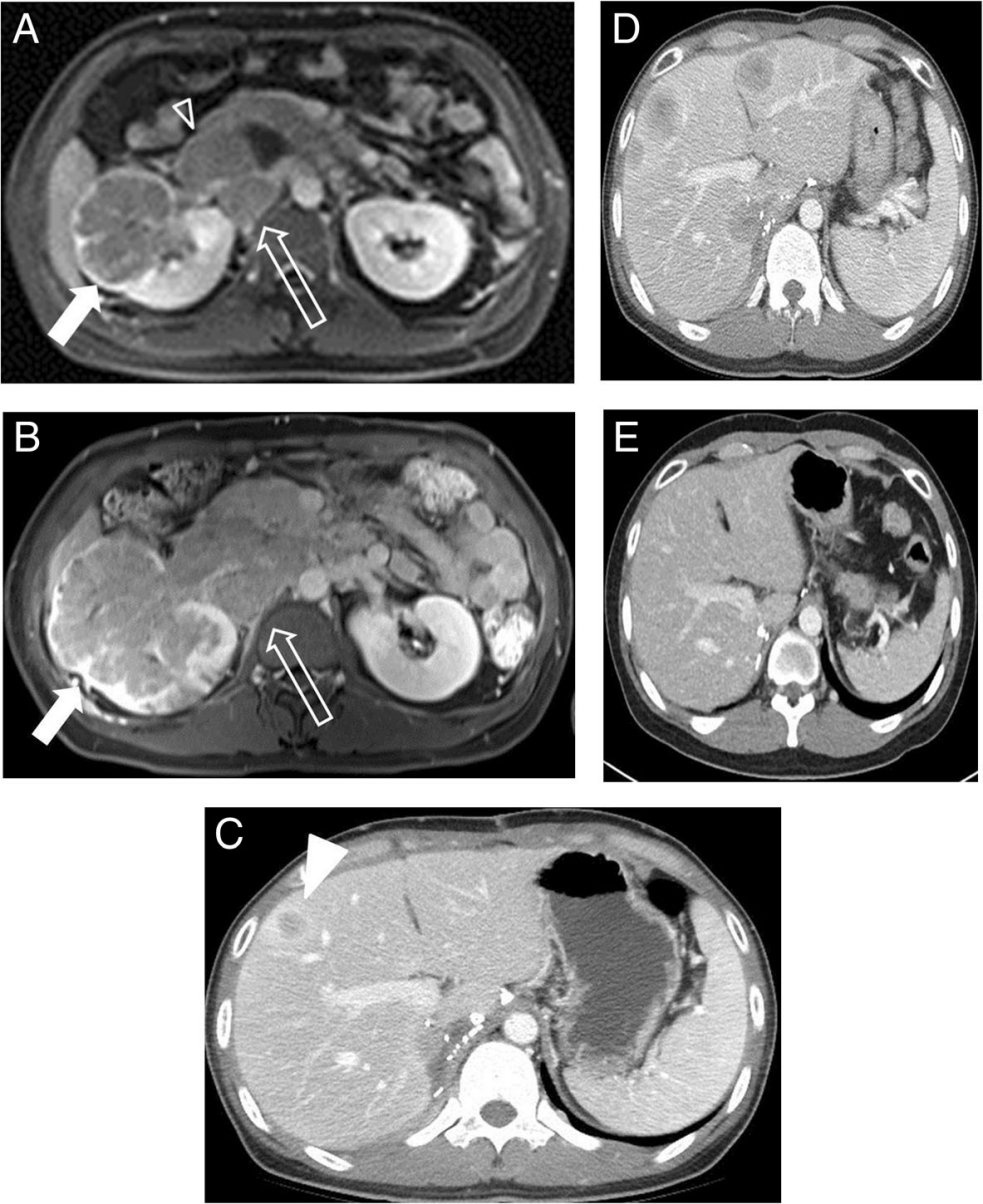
Serial CT and MRI images. a Axial fat saturated T1-weighted MRI image with contrast enhancement showing a lobulated contoured poorly enhancing tumor (arrow) in the right kidney. Tumor invasion was observed at IVC (arrow head) and metastatic lymph nodes (open arrow) were noted. b Despite temsirolimus treatment, primary tumor and metastatic lymph node size increased. c After radical nephrectomy, hepatic metastasis (arrowhead) was noted on a follow up CT scan, which (d) became aggravated despite axitinib therapy. e However, at 2 months after bevacizumab plus erlotinib therapy, the multiple hepatic metastases had nearly disappeared
